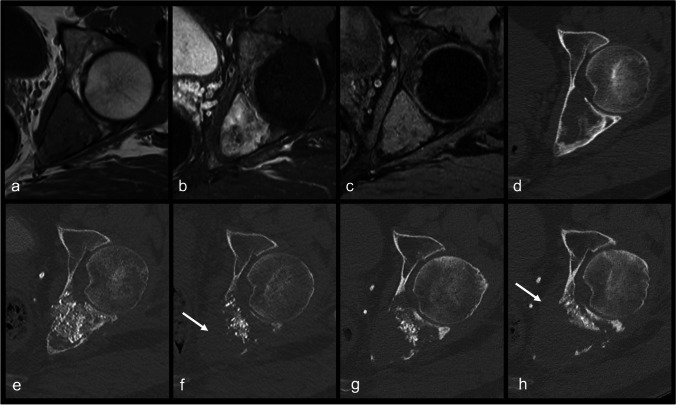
Imaging of the bone lesion at different stages of treatment. MR images (a, b, c) show a tumor located in the posterior wall of the acetabulum, which is isointense to muscle on T1-weighted sequences (a), hyperintense with slight heterogeneity on fat-suppressed T2-weighted sequences (b), and moderate enhancement on fat-suppressed post-contrast T1-weighted sequences (c). On CT (d), the lesion is well-defined, osteolytic, with a rim of sclerosis at the periphery and areas of cortical scalloping. Post-operative CT after curettage is shown (e). Three years after treatment, CT (f) shows diffuse bone lysis, as well as progression into soft tissues (arrow). One year after initiation of denosumab therapy, CT (g) shows a decrease in the tumor size and ossification notably close to the subchondral bone. About 2 years of the initiation of denosumab, CT (h) shows signs of tumor progression, with soft tissue extension (arrow) as well as bone lysis
